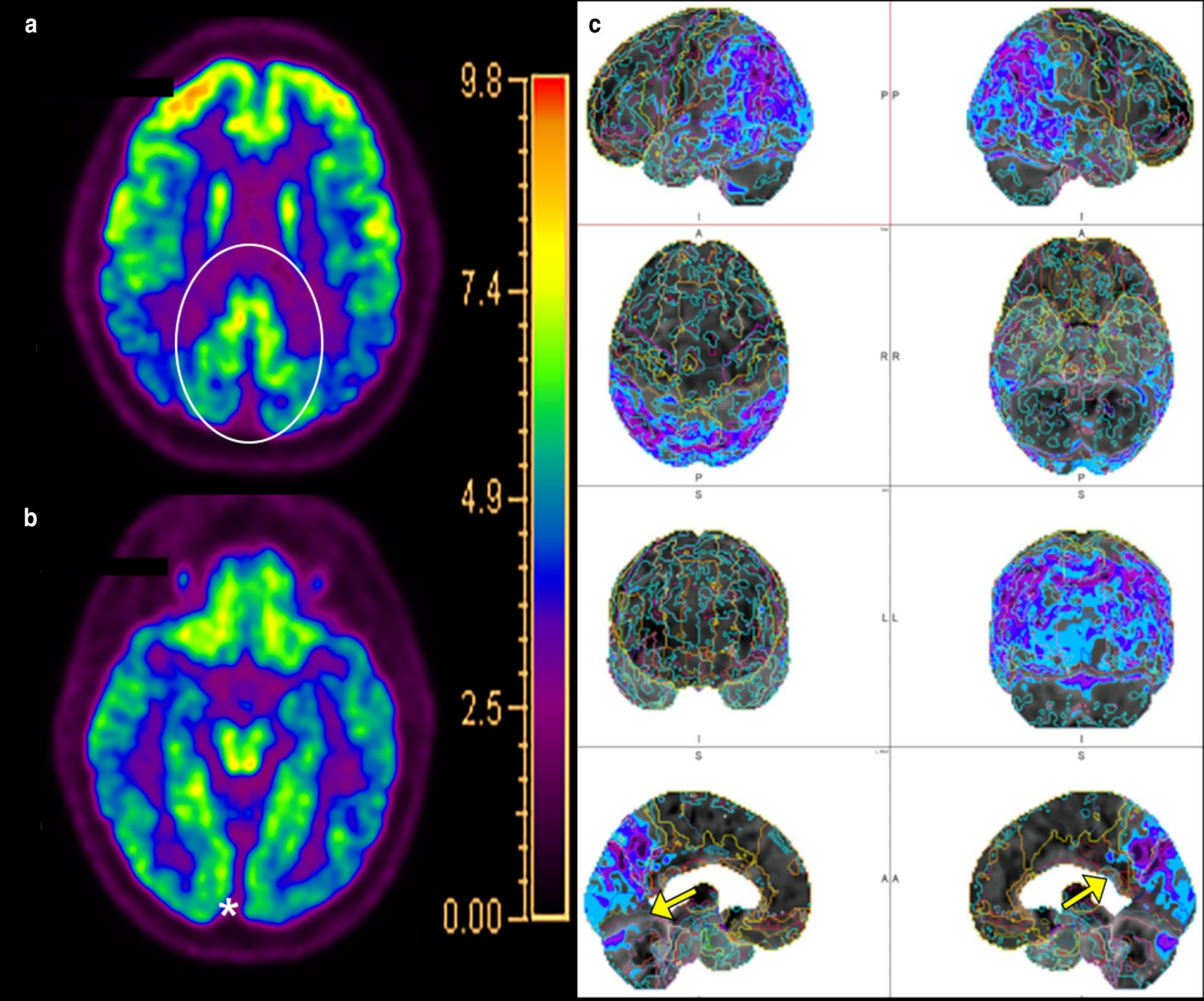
A 73-year-old man with memory loss and visuospatial processing deficits. Fluorodeoxyglucose PET/CT demonstrates hypometabolism in the bilateral parietal lobes including the bilateral precuneus with preserved metabolism of the PCC (cingulate island sign, oval in a). Also present is hypometabolism of the bilateral lateral occipital lobes with preserved medial occipital lobe activity (occipital tunnel sign, asterisk in b). Single-subject statistical parametric mapping (c) demonstrates parietal-occipital hypometabolism reiterating the occipital tunnel (arrow bottom left panel) and posterior cingulate island (arrow bottom right panel), with areas of blue progressing to purple representing further negative deviation from the mean uptake values of controls
